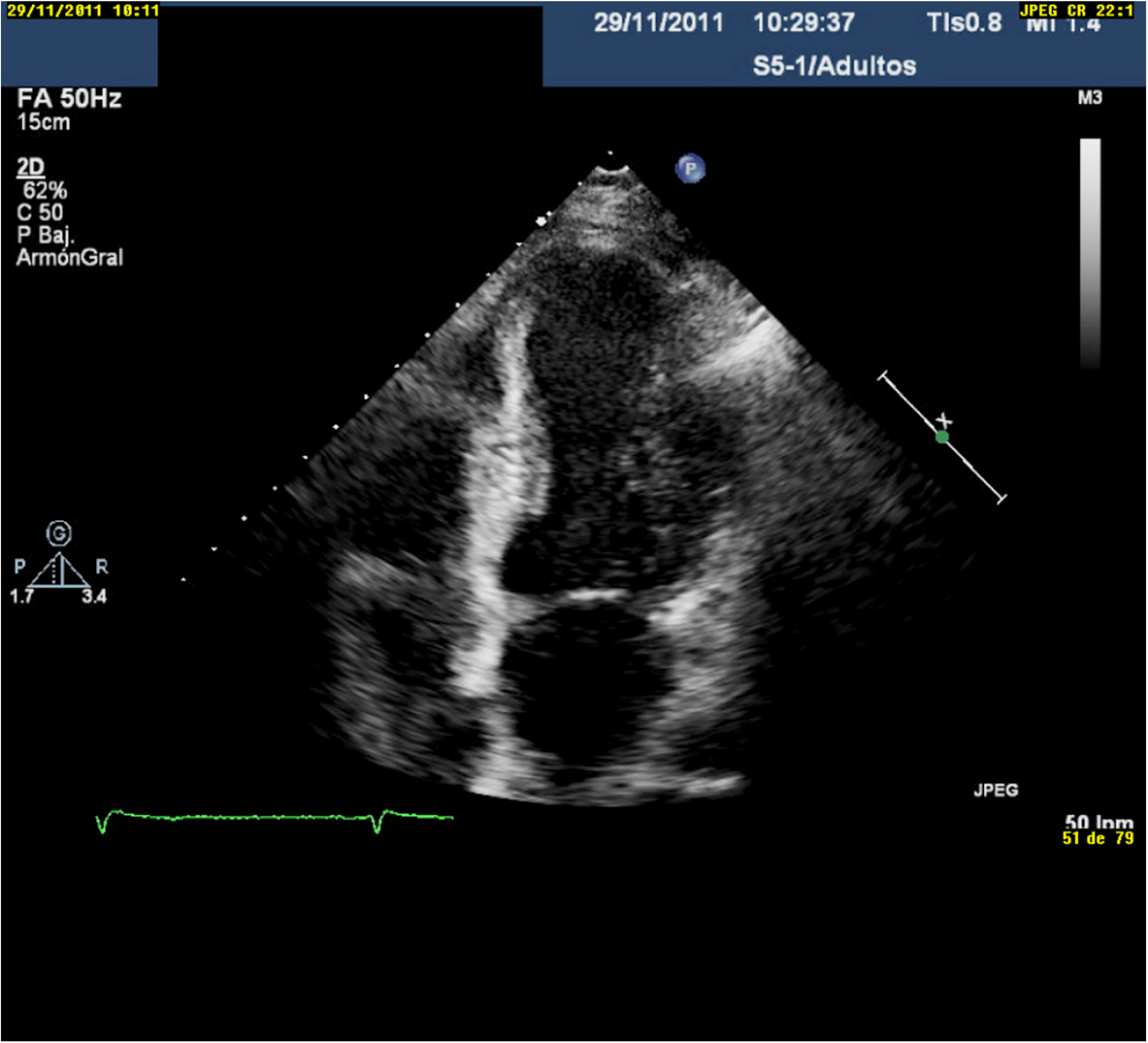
At 4 months after discharge, transthoracic echocardiography showed absence of left ventricular thrombus, persistence of apical-anterior and anterior akinesis, and resolution of the abnormal contractility in the area supplied by the revascularized right coronary artery.
